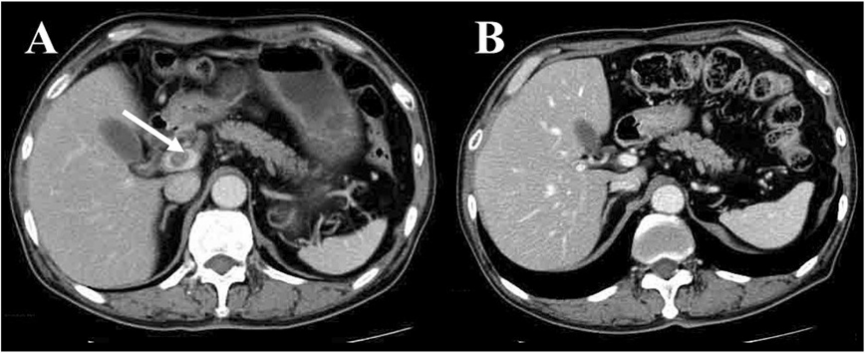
Abdominal computed tomography imaging. (A) Before chemotherapy with S-1 plus cisplatin (B) After 3 courses of chemotherapy.
